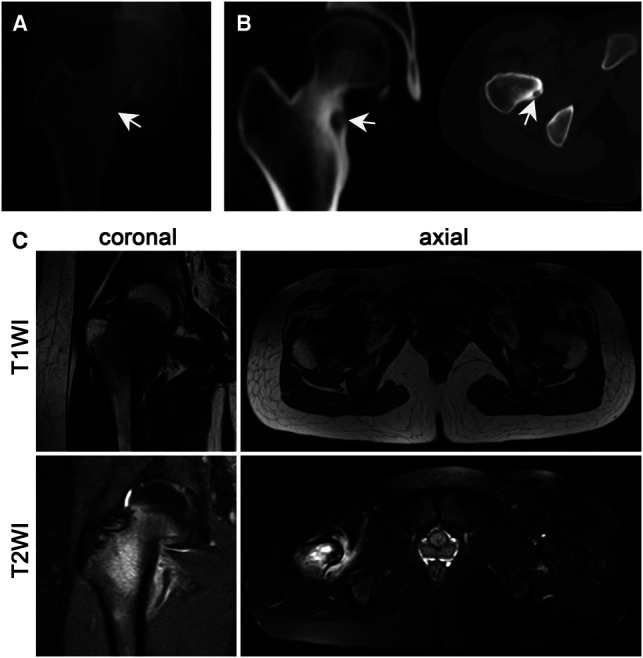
Imaging examination significantly helped in the diagnosis of OO. (A) The radiography examination shows a round and transparent area. (B) Thin-layer CT accurately displays the nidus and the surrounding reactive hyperplastic bone tissue (the white arrow shows the nidus). (C) MRI examination lacks specificity, T1WI shows low to moderate signal intensity and T2WI shows moderate to high signal intensity.
